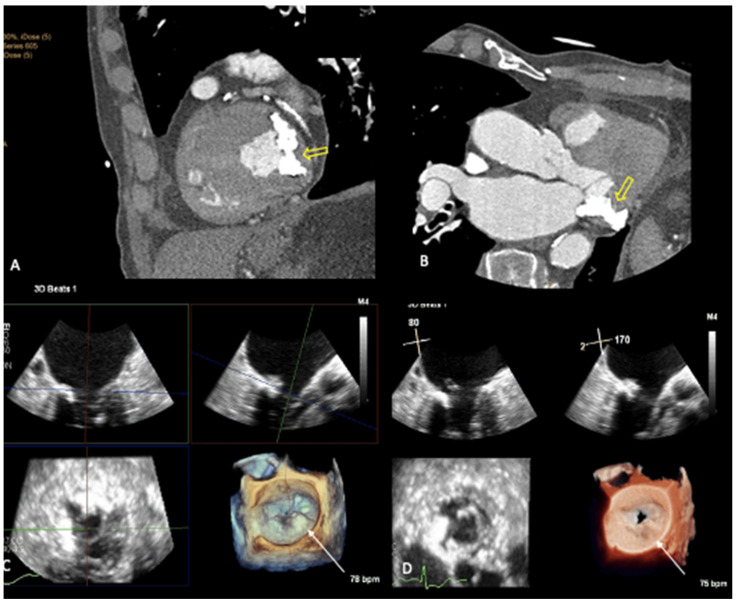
Cardiac computed tomography scan from a patient with severe circumferential mitral annular calcification (MAC) with caseous necrosis extending posteriorly beyond the annulus (A,B, yellow arrows). Transesophageal echocardiogram (TEE) from the same patient, documenting MAC in two-dimensions (2D) and mitral annular irregularity in three-dimensional (3D) volume-rendering colorized-depth map (C, white arrow) and transillumination map (D, white arrow).
